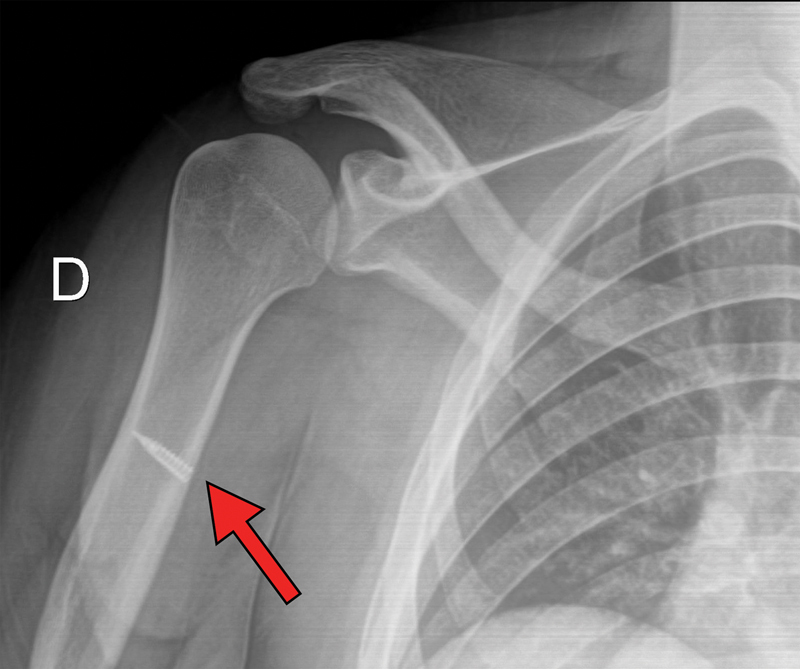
Follow-up radiograph of the position of the metallic anchor (red arrow).
